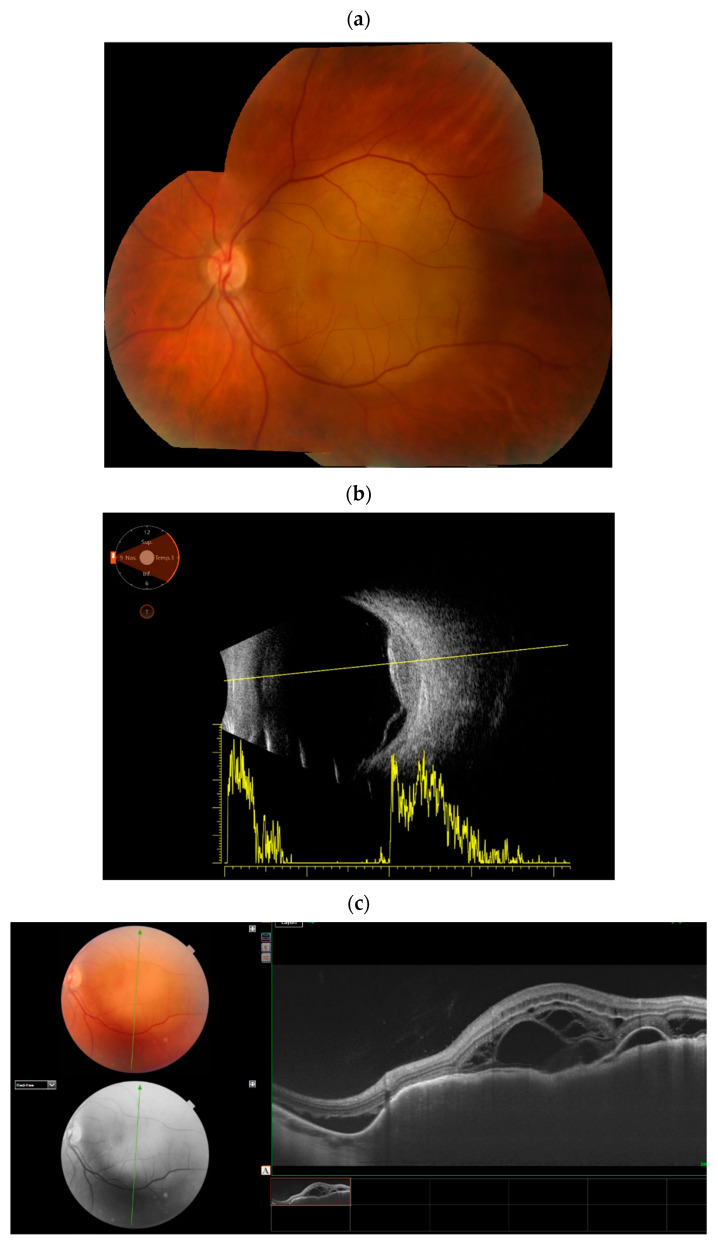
Choroidal metastasis in the left eye of a 37-year-old woman with breast cancer: color fundus photography (a) and ultrasound (b) showing a lesion with medium internal reflectivity and serous retinal detachment; OCT (c) demonstrates an irregular “lumpy bumpy” anterior contour of the tumor, subretinal fluid, and retinal pigment epithelium abnormalities.
